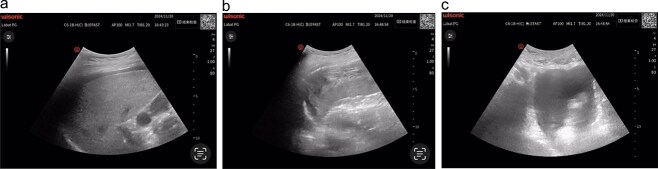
(a) Hepatic ultrasonography demonstrates an intact hepatic capsule with adjacent anechoic fluid collections; (b) splenic imaging reveals discontinuous capsular contour at the diaphragmatic surface with heterogeneous parenchymal echotexture; (c) pelvic cavity shows significant anechoic fluid accumulation.
